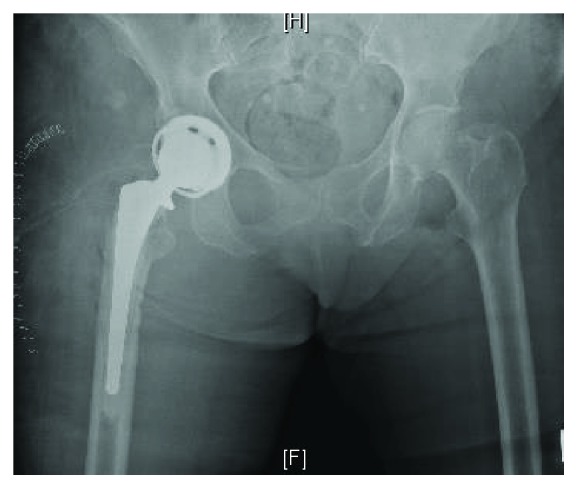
Anteroposterior (AP) pelvis radiograph, status post right hip irrigation and debridement, greater trochanter excision, hook plate, and cable removal of hardware.
